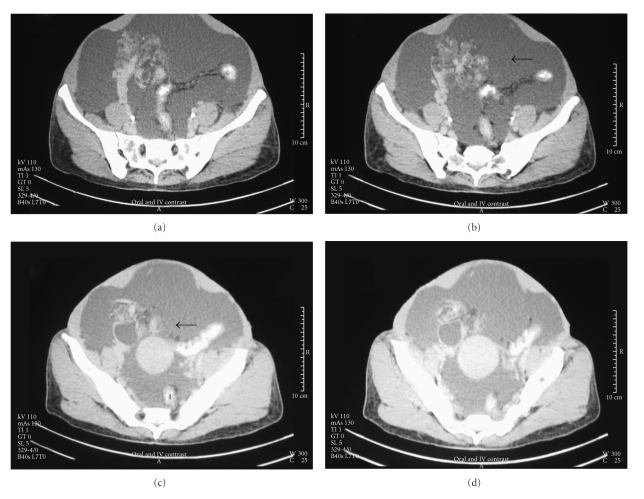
CT Scan—Heterogenous, solid-cystic, moderately enhancing right adnexal mass (↑).
